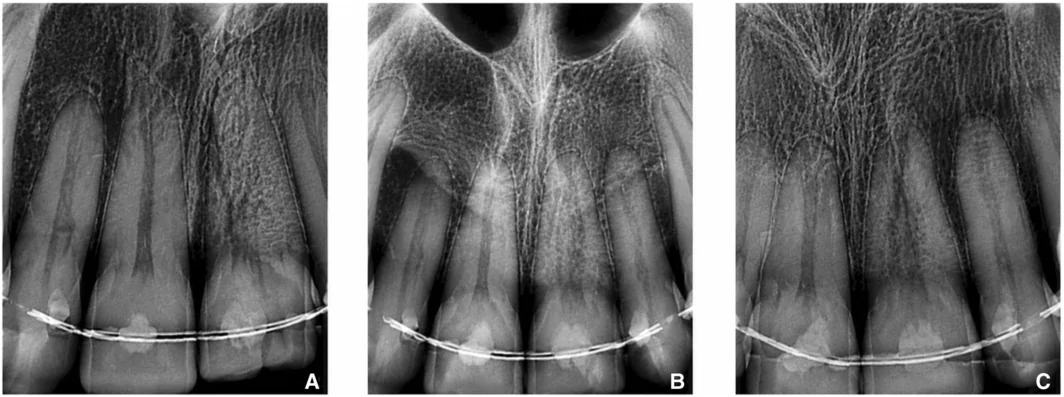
Preoperative intraoral radiographic examination of the maxillary anterior region, obtained on October 30, 2017. (A) Lateral intraoral radiograph of the left maxillary anterior region. (B) Intraoral radiograph of the maxillary anterior region. (C) Lateral intraoral radiograph of the right maxillary anterior region.
